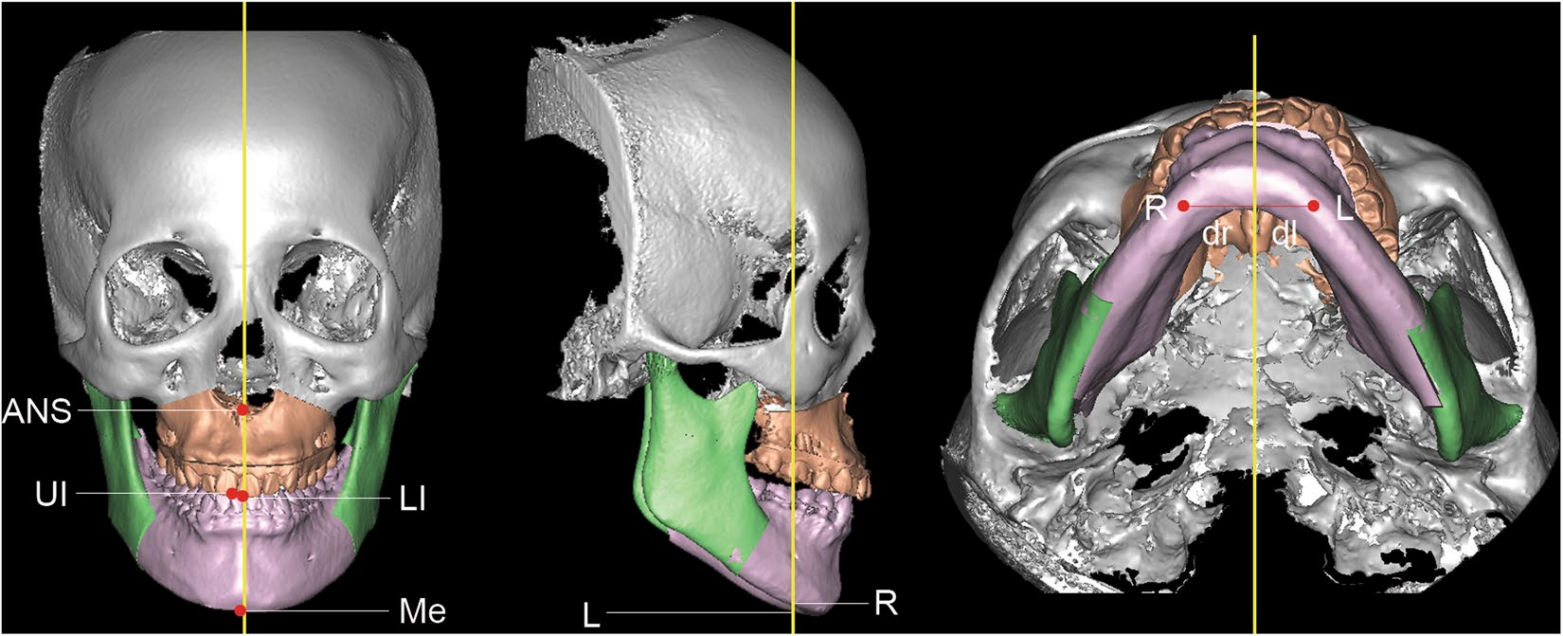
Computed tomography images used for linear and angular measurements of jaw symmetry. (Left) Horizontal deviation for maxilla (anterior nasal spine and upper incisor) and mandible (lower incisor and menton); ANS, anterior nasal spine; UI: upper incisor; LI: lower incisor; Me: menton. (Center) Vertical discrepancy for mandible (distance between point R and point L); point R: inflection point of mandibular body at the level of right mental foramen; point L: contralateral corresponding point of mandibular body on the left side. (Right) Horizontal discrepancy for mandible (distance discrepancy between dr and dl); dr: distance from point R to the facial midsagittal plane; dl: distance from point L to the facial midsagittal plane.
